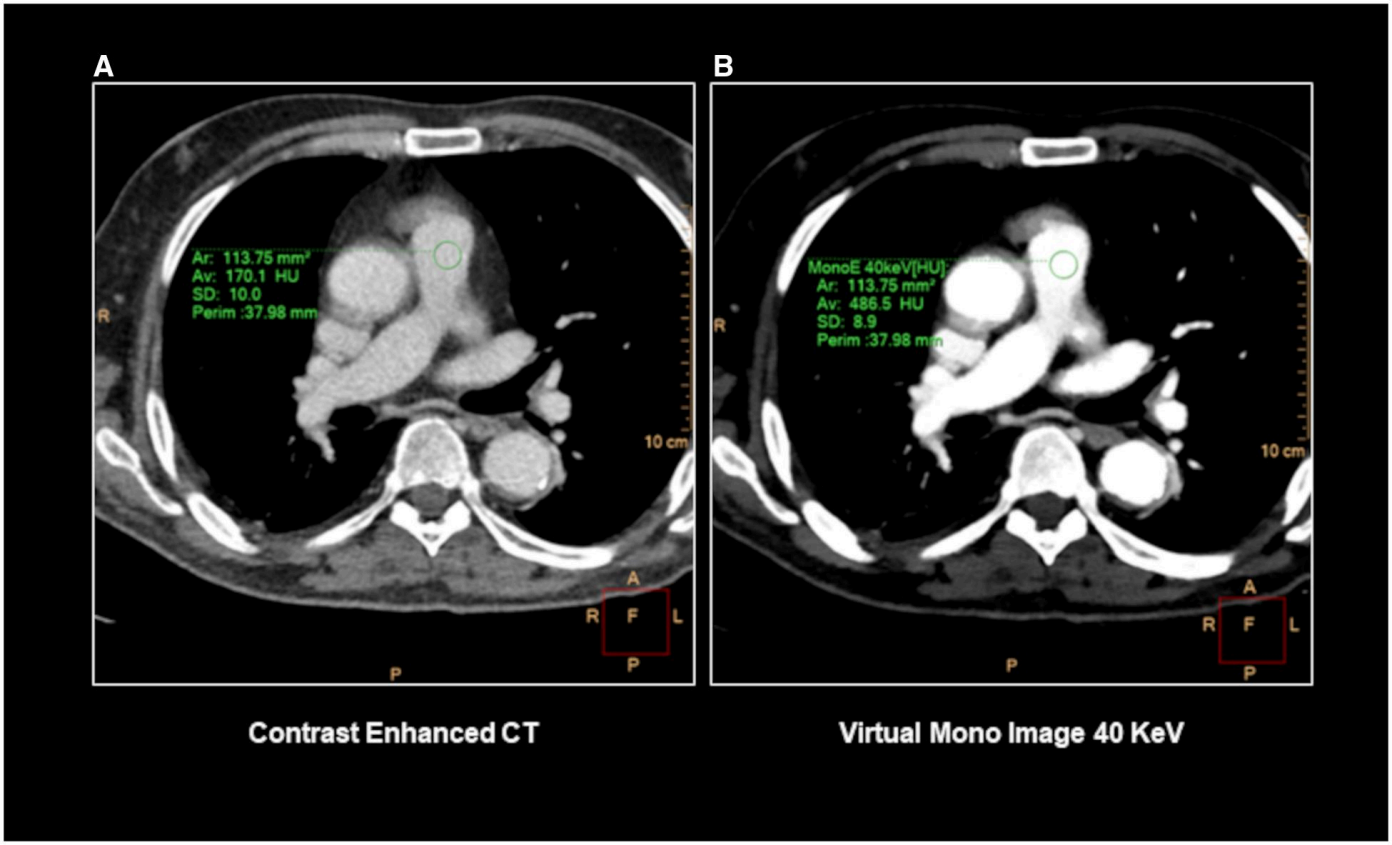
Example of conventional CECT (contrast-enhanced computed tomography) of the chest with poor contrast attenuation of pulmonary vessels (A). The use of VMI (virtual monoenergetic images) reconstruction at 40 keV (B) allows for increasing vascular attenuation (ie, 486 HU at the level of main pulmonary artery).
